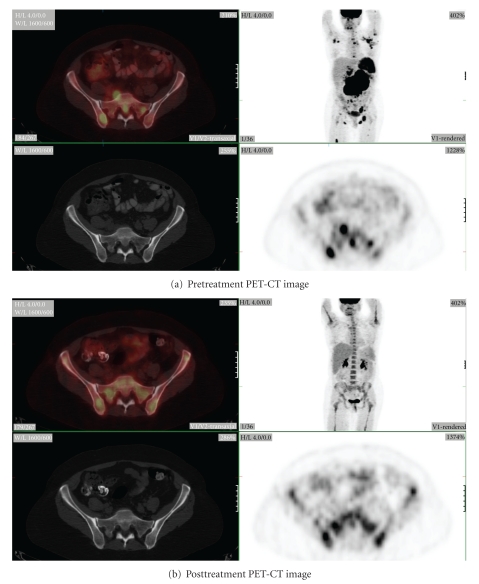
53-year-old female with follicular grade 3 lymphoma with clear marrow involvement (a). This patient had bilateral iliac marrow biopsy. The post-treatment PET-CT (b) shows diffuse uniform increase in marrow uptake. However, the SUV actually decreases (from 6.7 to 4.9) on the right iliac bone marrow with pathologic confirmation of marrow involvement (b). On the contrary, the SUV over the pathologically negative left iliac bone marrow increases (from 2.7 to 4.2) due to normal hematopoietic response.
